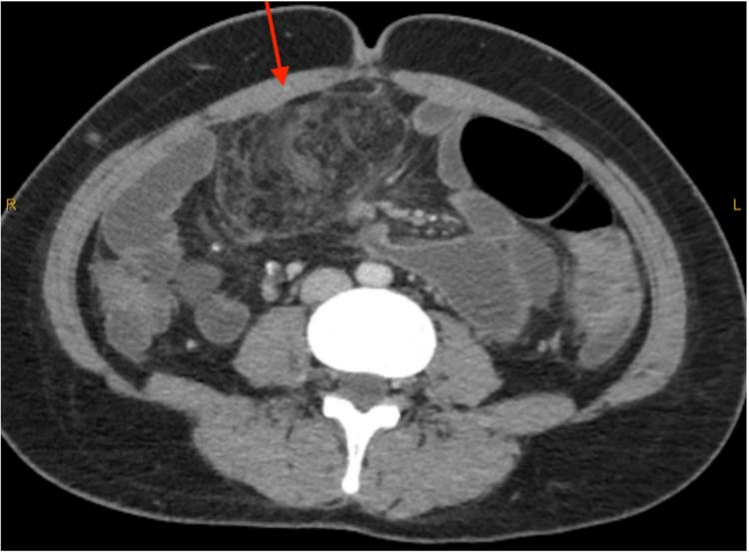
CT abdomen pelvis in 2023 (axial).
